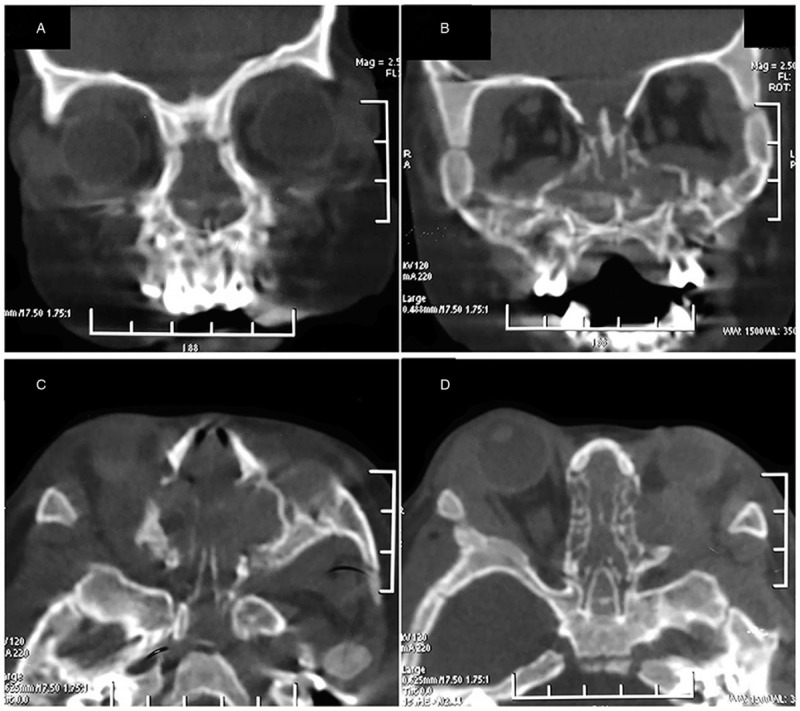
CT scan findings. Bilateral nasal cavity was full of tissue mass (A, B: Coronal view; C, D: Axial view). CT = computed tomograpghy.
